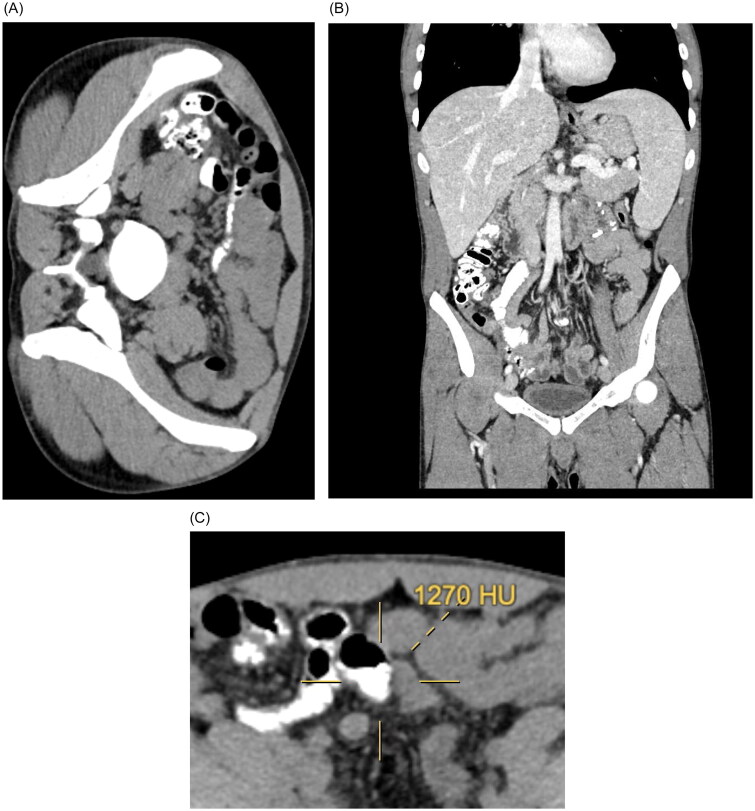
Computed Tomography (CT) Scan Demonstrating Radiopacity in the Bowel due to Sodium Zirconium Cyclosilicate (SZC). Panel A: Axial non-contrast CT image of the abdomen showing heterogeneous high-density material within the lumen of the small intestine, especially in the distal ileum. The material presents a radiopacity comparable to oral contrast medium but without prior administration. The image highlights the gravitational layering effect of the radiodense material. Panel B: Coronal non-contrast CT image of the abdomen and pelvis again demonstrating the high-density material distributed throughout the bowel lumen. Panel C: Highlight of the Hounsfield units (mean 1270 HU).
